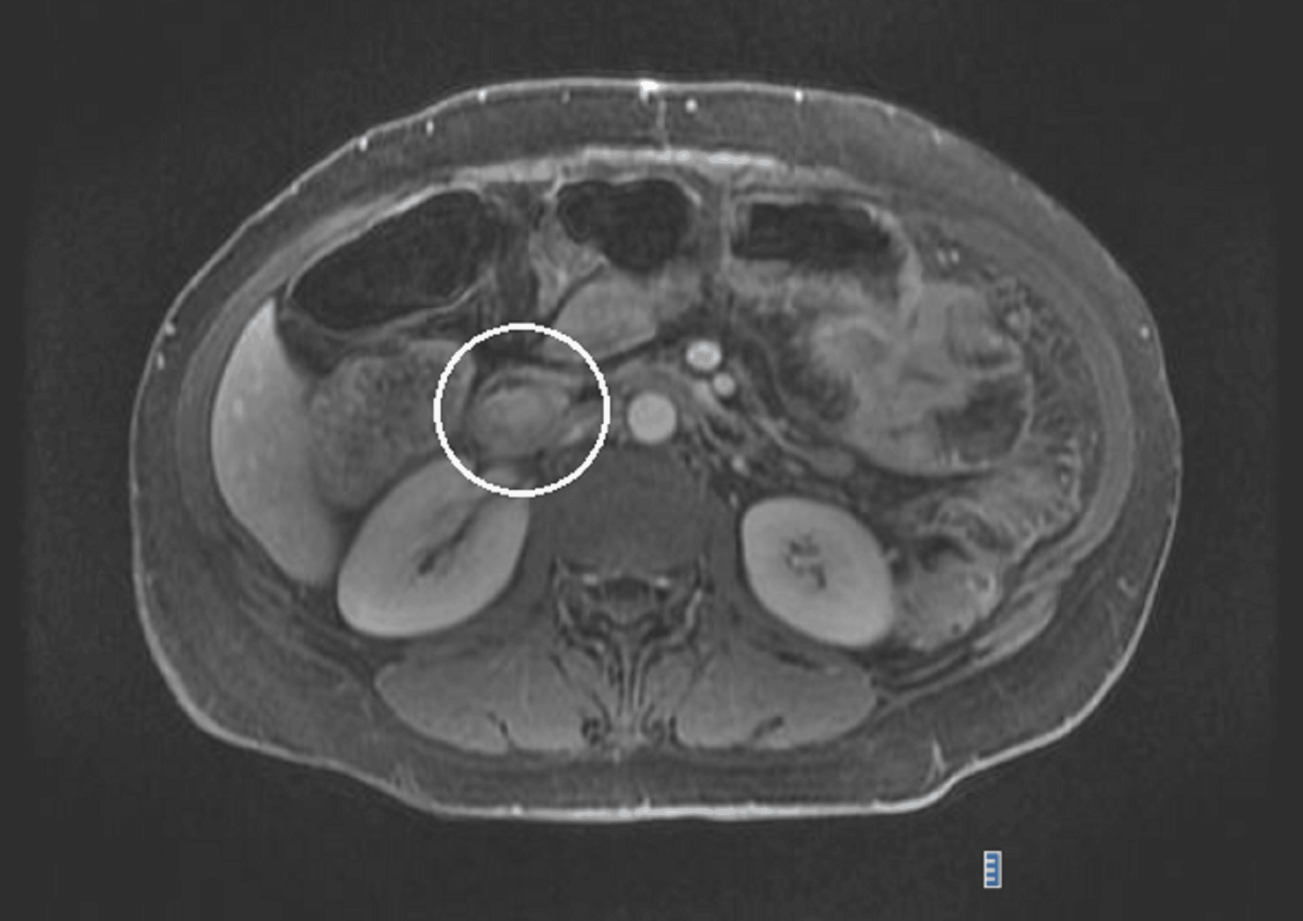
MRI of the upper abdomen MRI showing a well-defined, intraluminal polypoid lesion in the second portion of the duodenum adjacent to the papilla, measuring 3.2 cm in its greatest dimension. The lesion demonstrates heterogeneous enhancement, suggestive of vascularity, with no evidence of invasive features. Associated biliary dilatation is also observed, indicating possible obstruction. The distinct borders and absence of tissue invasion help differentiate this lesion from more aggressive malignancies. MRI: magnetic resonance imaging
